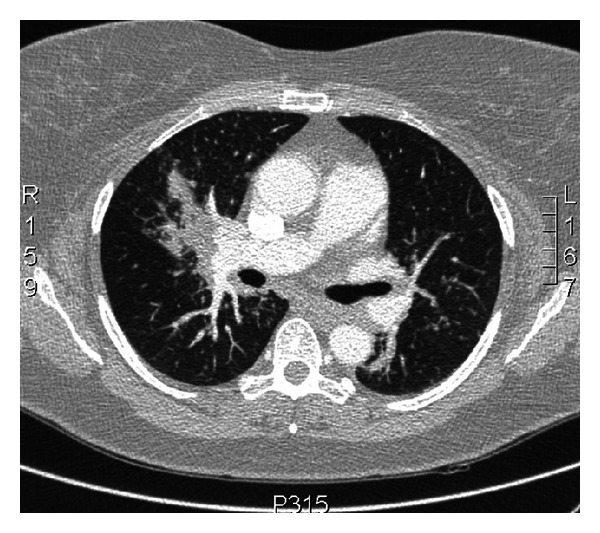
HRCT of the thorax after discontinuation of infliximab showing increased consolidation and nodular abnormalities.
